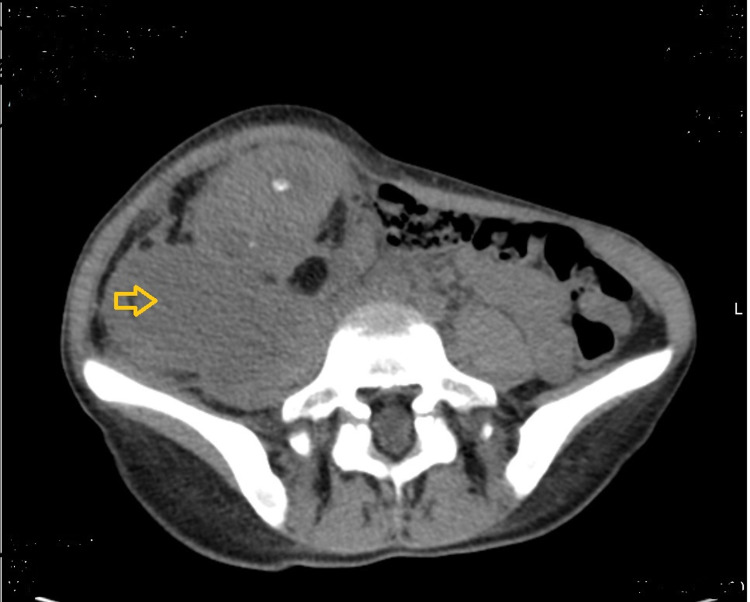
Abdominal and pelvic CT scan demonstrating psoas abscess collection
